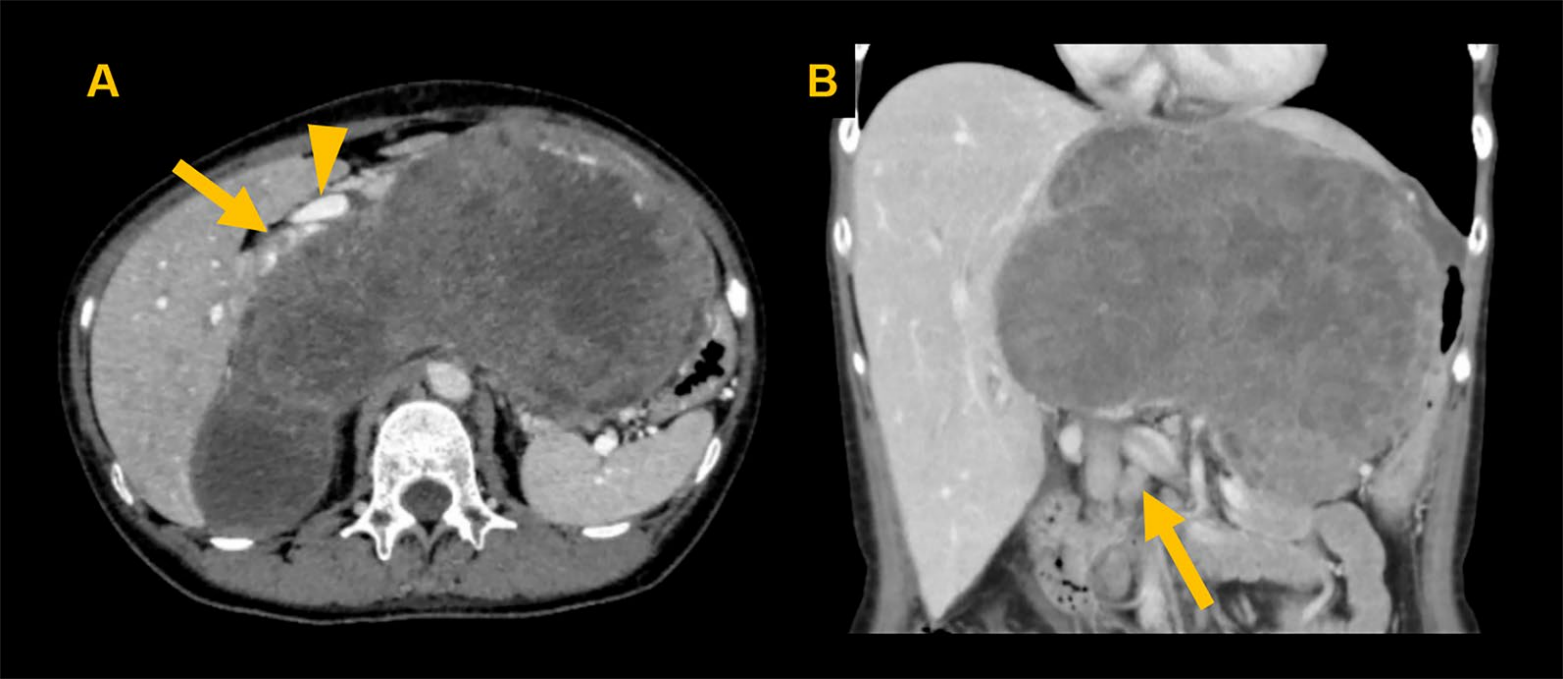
Preoperative imaging findings. Contrast-enhanced CT shows a hemorrhagic retroperitoneal tumor (22 × 9 × 15 cm) compressing the IVC (arrow) and portal vein (arrowhead) (A) and an enlarged para-aortic lymph node (arrow) (B)
